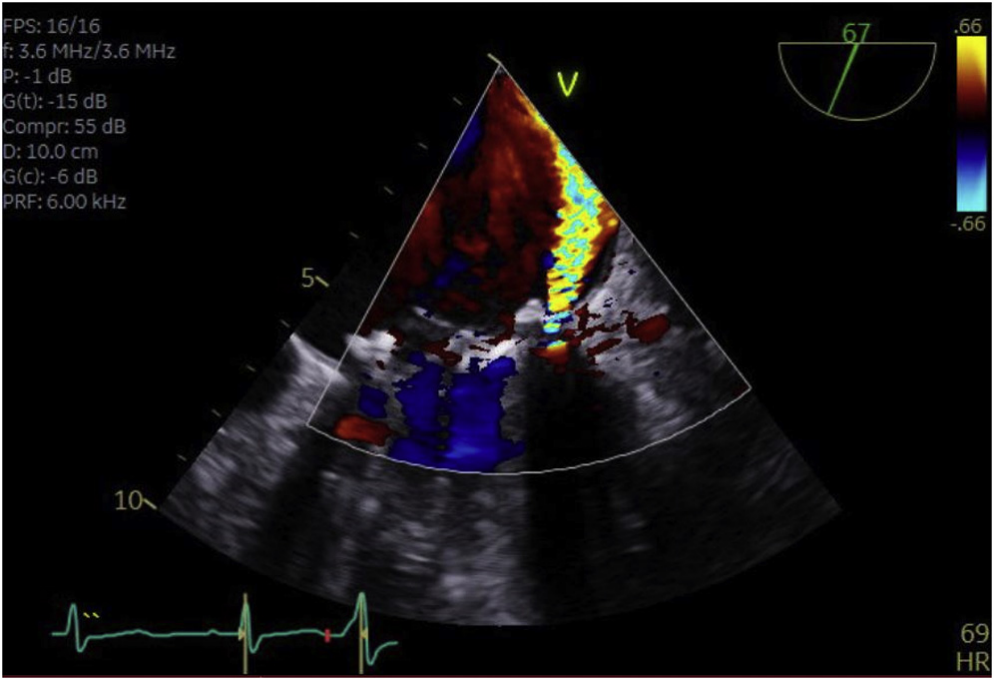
Transesophageal echocardiography: Anterolateral mitral paravalvular leak.
